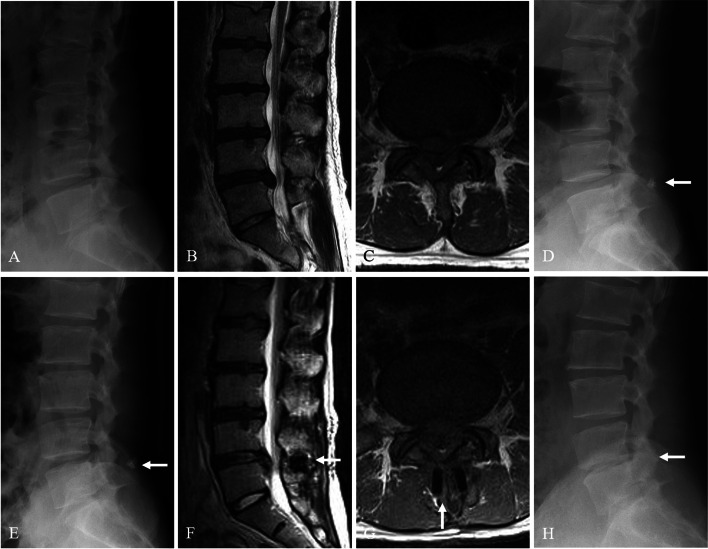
A 36-year-old woman with lumbar disc herniation (LDH) at L4–5. Lateral (A) radiographs showing lumbarization of S1, becoming L6. Magnetic resonance images (B and C) showing LDH at L5–6. The patient underwent discectomy and DIAM implantation at L5–6 (white arrow) (D). At 8 months after surgery, lateral radiograph (E) and magnetic resonance images (F and G) showing maintenance of DIAM (white arrows) but recurrent LDH at L5–6. At 5 years after DIAM removal and another discectomy, lateral radiograph (H) shows disc space narrowing at L5–6 (white arrow)
